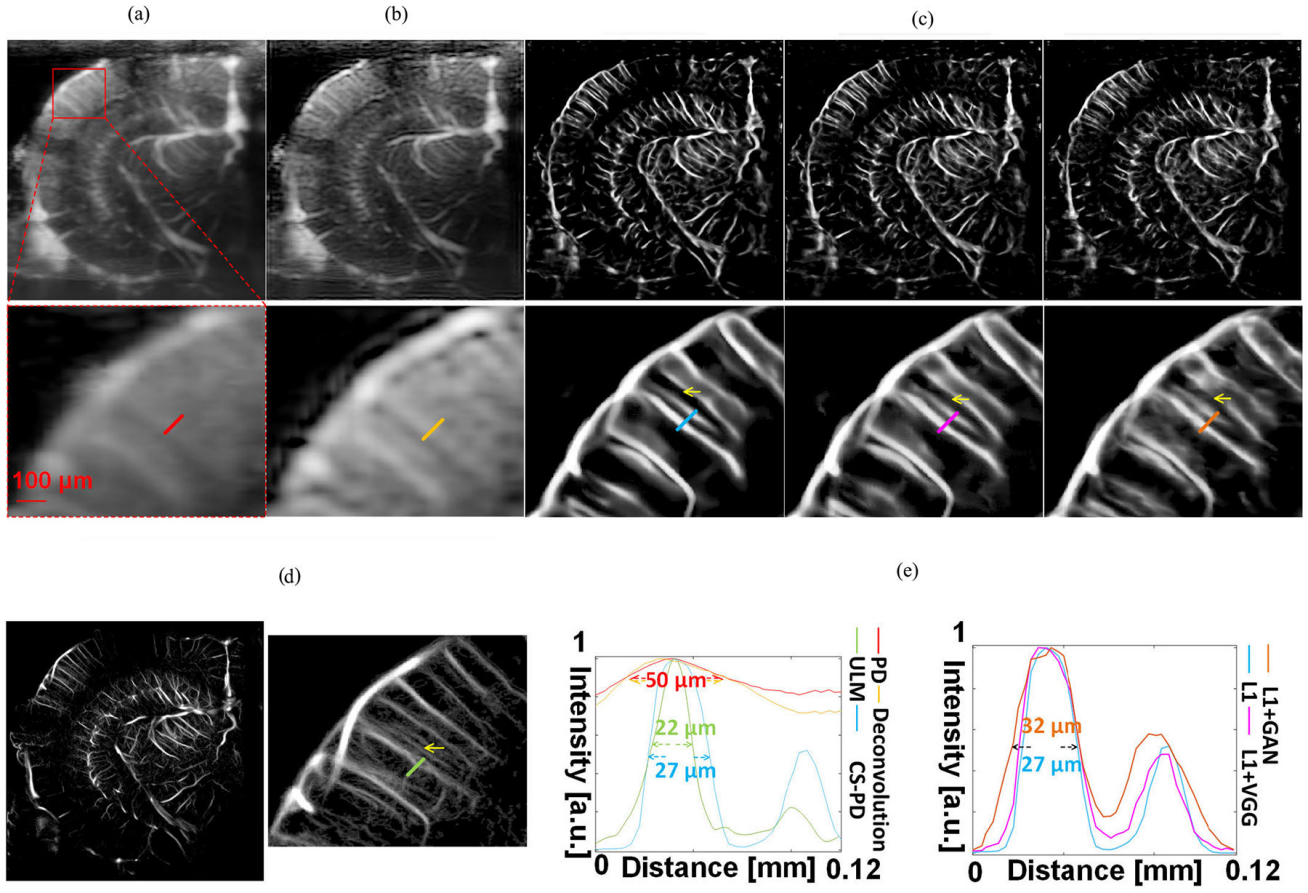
(a) Conventional power Doppler image accumulating 400 frames of contrast-free ultrasound data. (b) Deconvolution-based power Doppler image reconstructed using 400 frames of contrast-free ultrasound data. (c) CS-PD images using different loss functions reconstructed using 400 frames of contrast-free ultrasound data. (d) ULM image reconstructed using 32 000 frames of contrast-enhanced ultrasound data. (e) Intensity profile of the small penetrating cortical vessels marked by the short line segments with different colors. All the intensity profiles were normalized in the linear scale. All the zoomed-in local vessel images (bottom row) correspond to the same region marked by the red box in the power Doppler image (a).
